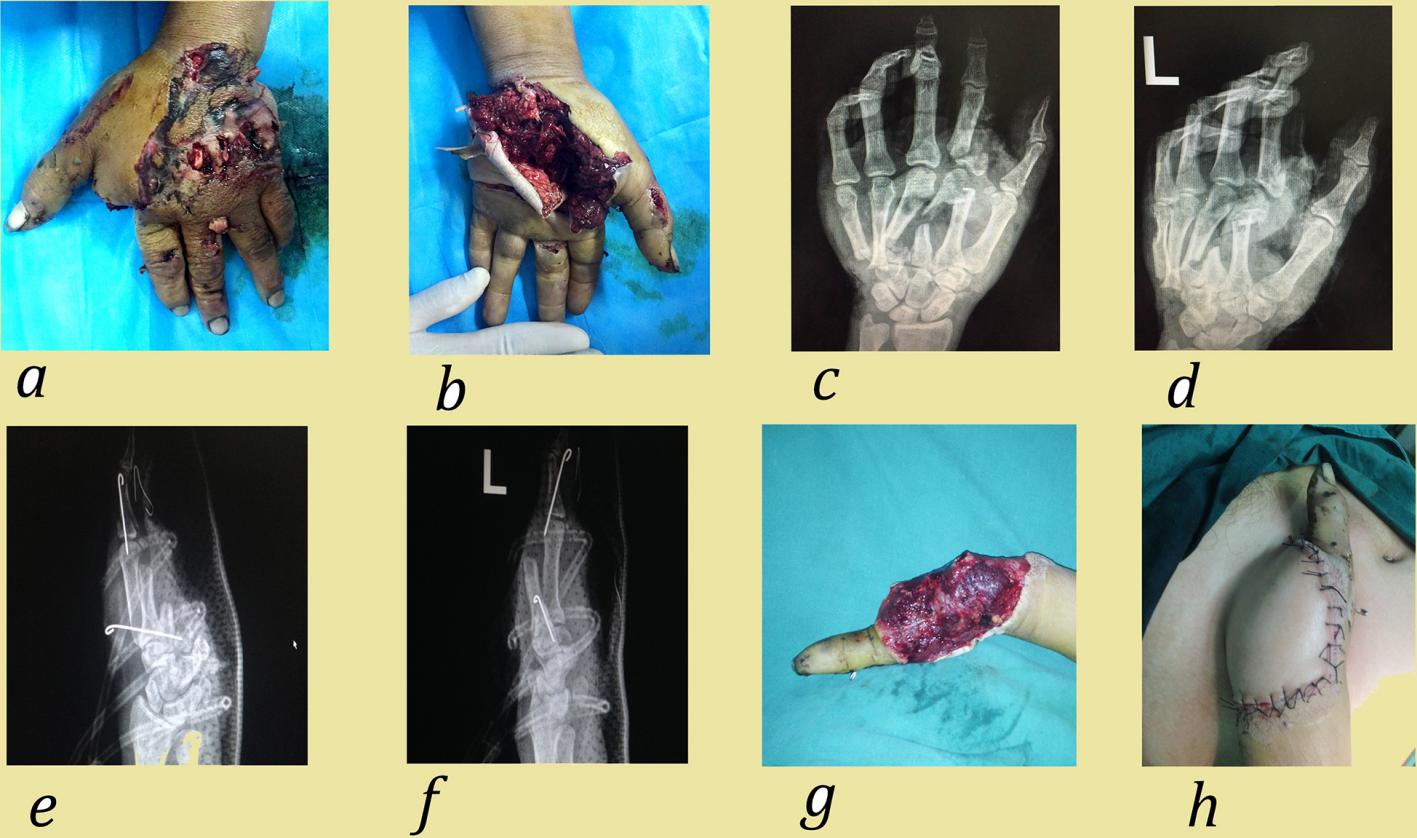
Case 2. A 34-year-old man suffered corn-husker machine injury to his left hand involving multiple metacarpal fractures, neurovascular and tendon injuries. (a,b) Preoperative view; (c,d) Preoperative positive and lateral radiograph; (e,f) Post operative positive and lateral radiograph. The emergency surgical methods include: debridement, fracture reduction, K-wire fixation, amputation (index finger, middle finger, ring finger, little finger and the palmar ulnar side) and NPWT treatment; (g,h) Appearance 1 week after the operation. After NPWT, granulation tissue grew and abdominal wall flap was performed.
